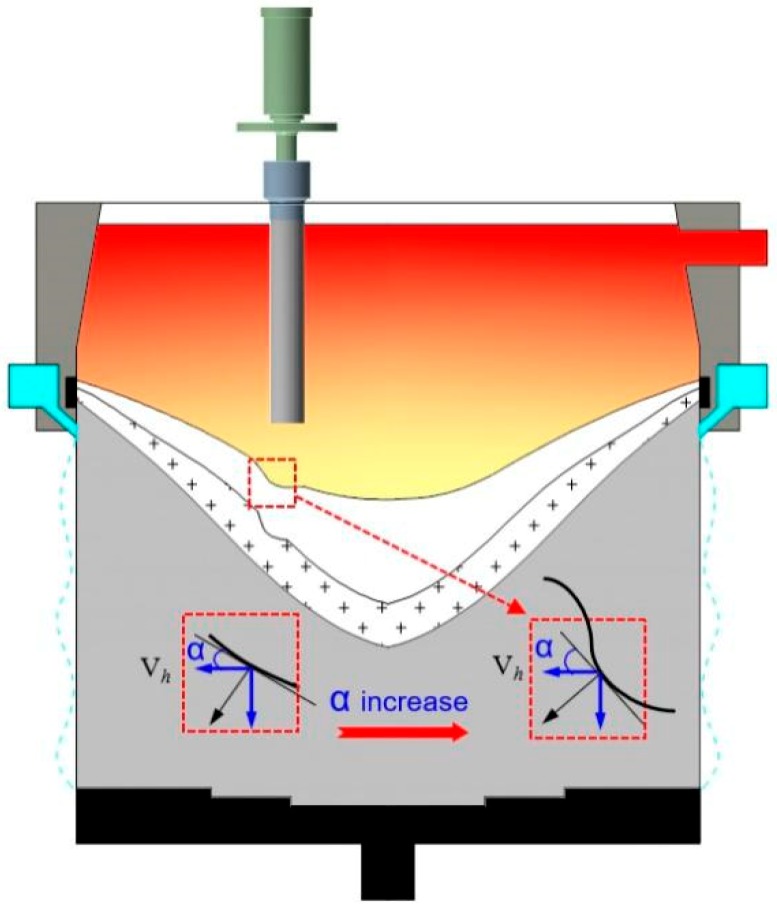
Effect of ultrasonic changes on the solidification front.
